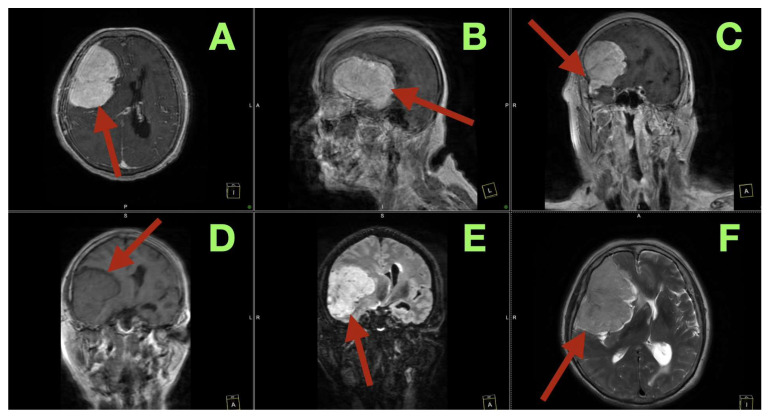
Preoperative MRI of the giant right sphenoid wing meningioma: (A) Axial T1 post-contrast image showing a large, homogenously enhancing right fronto-temporo-basal extra-axial mass (arrow) with broad sphenoid wing attachment, CSF cleft, compressed cortical ribbon, slit-like right frontal horn, and leftward midline shift. (B) Sagittal T1 post-contrast image demonstrating the full craniocaudal and anteroposterior extent of the tumor over the right frontal convexity, with frontal lobe flattening and bowing of the corpus callosum. (C) Coronal T1 post-contrast image highlighting basal dural implantation on the sphenoid wing/fronto-orbital floor and superomedial displacement of the insular–opercular cortex with deformation of the right lateral ventricle. (D) Posterior coronal T1 post-contrast image showing medial indentation of the cingulate gyrus and callosal body, consistent with subfalcine displacement. (E) Coronal FLAIR image demonstrating extensive vasogenic edema in the right frontal white matter and periventricular zones along frontal projection pathways. (F) Axial T2 image depicting the steep tumor–brain interface, broad edema rim, effaced right frontal sulci, and pronounced right-to-left midline shift.
